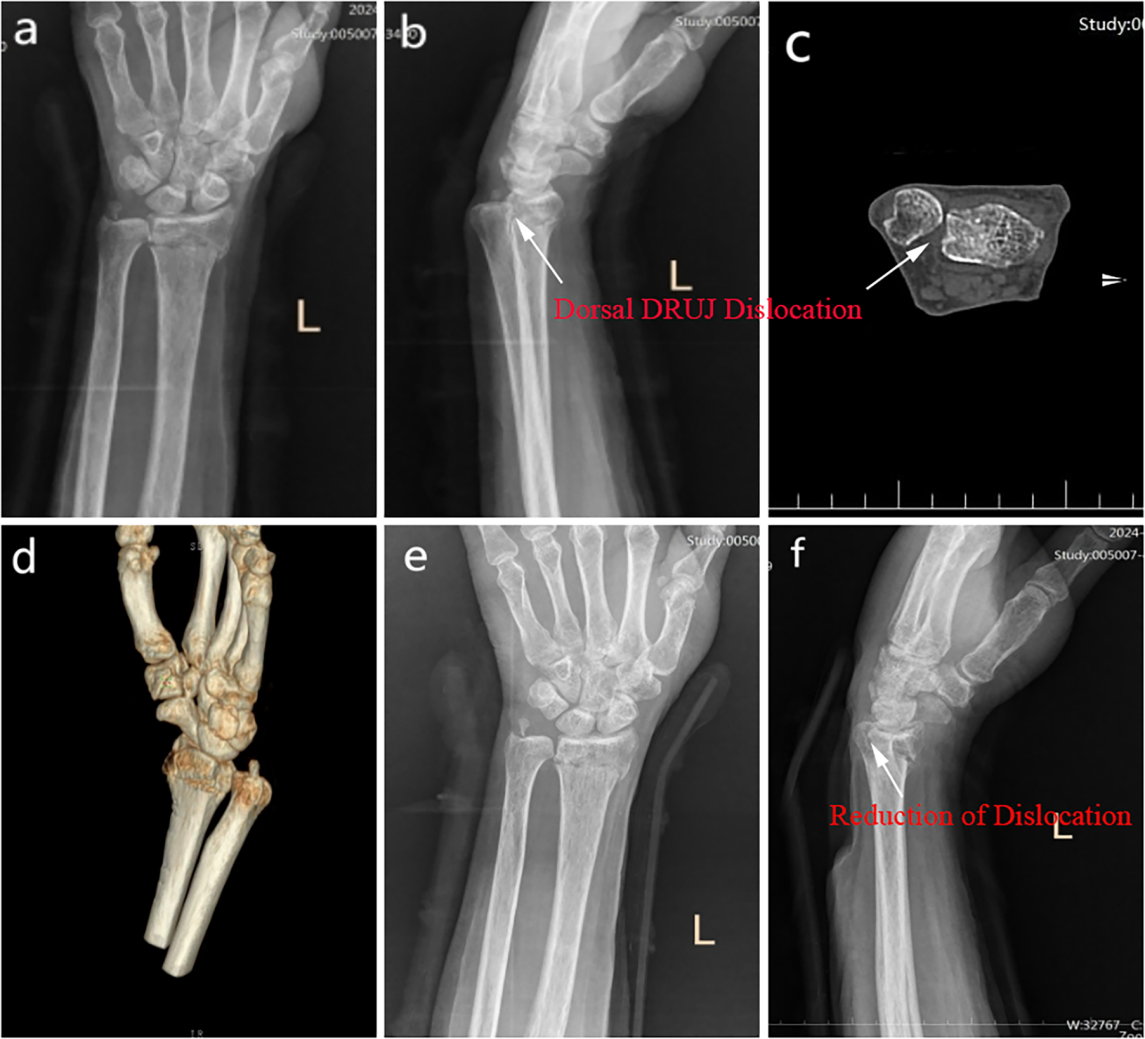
Drs and CT, and 3D reconstruction at the four-week follow-up. (a–d) no significant changes in the radius compared to previous imaging, with marked dorsal ulnar dislocation of the DRUJ. (e–f) the DRUJ is well-reduced, and the spacer is appropriately positioned. DR, digital radiograph; CT, Computed Tomography; DRUJ, distal radioulnar joint.
